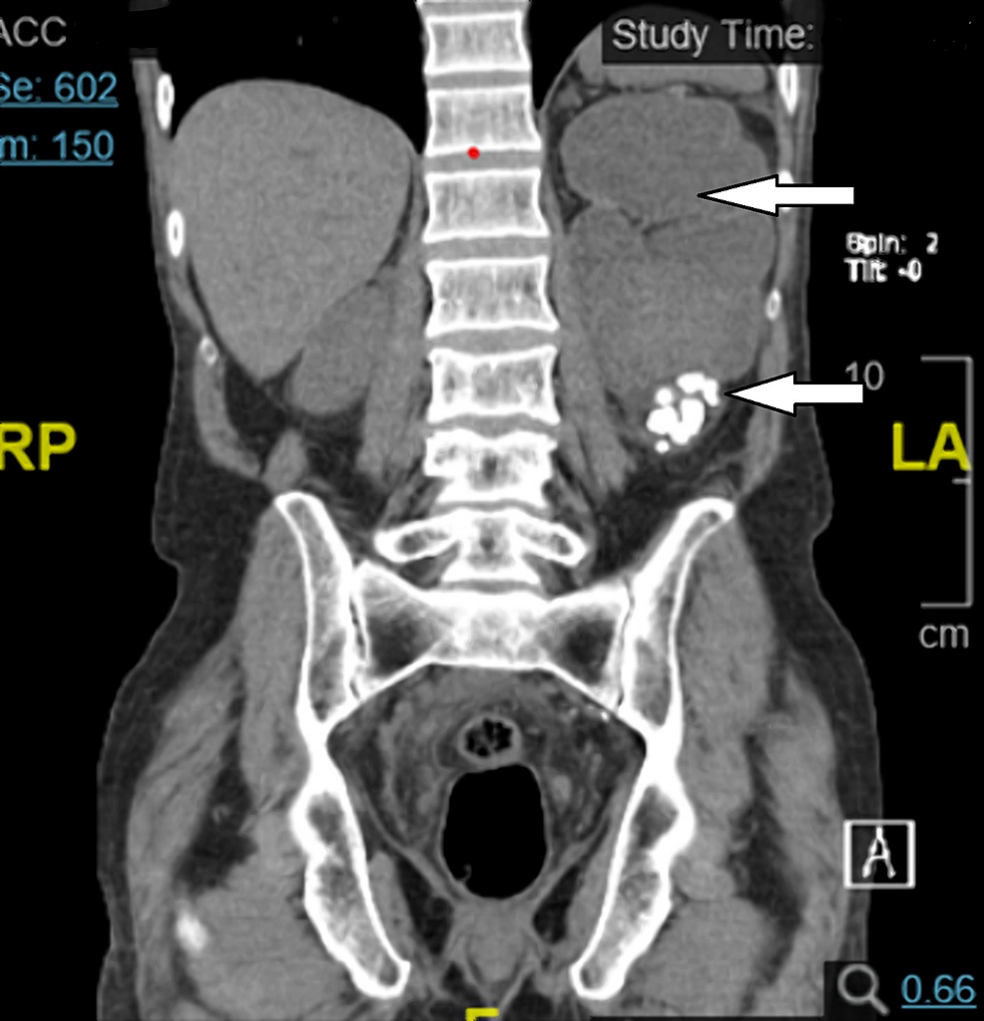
Coronal CT view of abdomen and pelvis displaying severe left hydronephrosis with multiple stones. CT: computed tomography.
